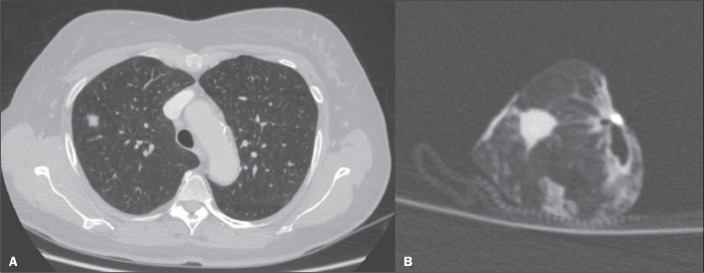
A: Axial CT scan of a 58-year-old female patient, showing a solid
